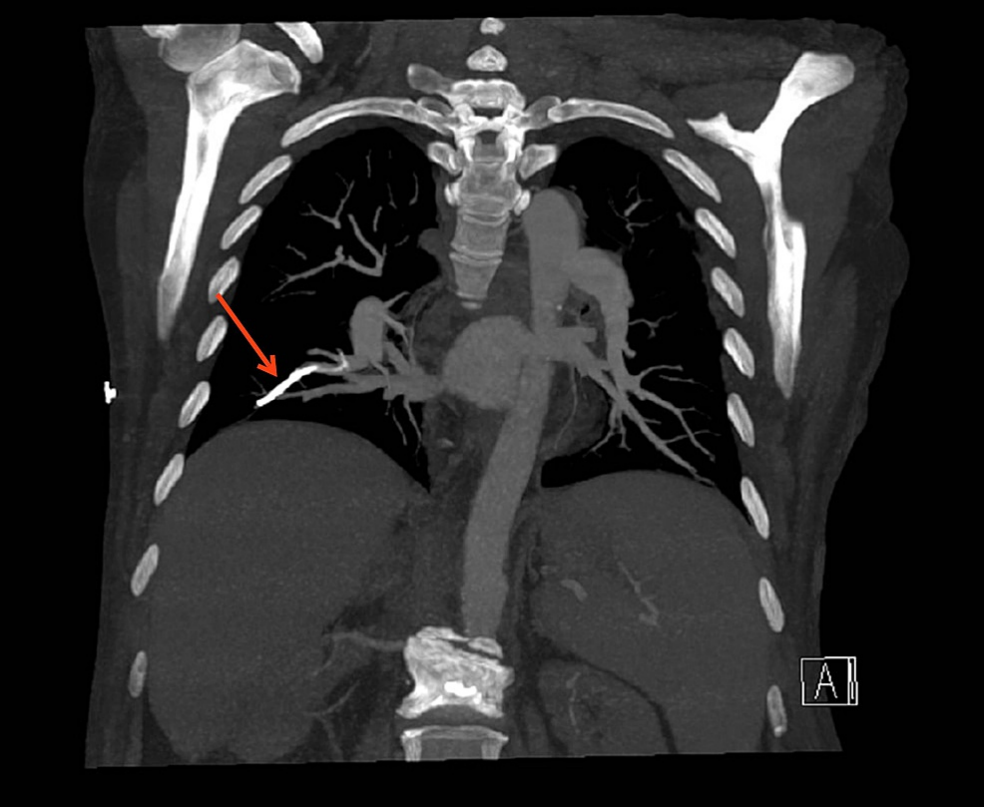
CTA of chest showing high density material in the vertebral column and subsegmental pulmonary artery of the right lower lobe. CTA: computed tomography angiography
